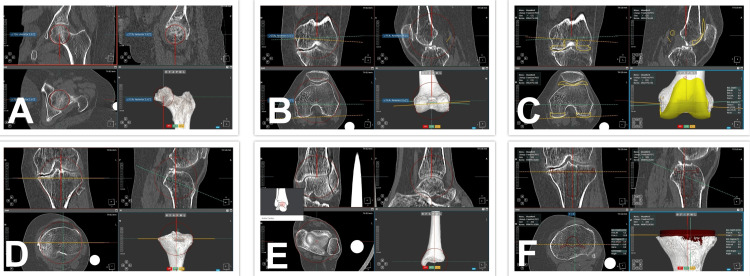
CT images of the hip, knee, and ankle in different planes analysed using the cuvis J Planner software to plan component size and positioning. A: center of the femoral head, B: intercondylar center with the surgical epicondylar axis, C: femoral component planning, D: proximal tibial center, E: centre of the ankle joint, F: tibial component planning
